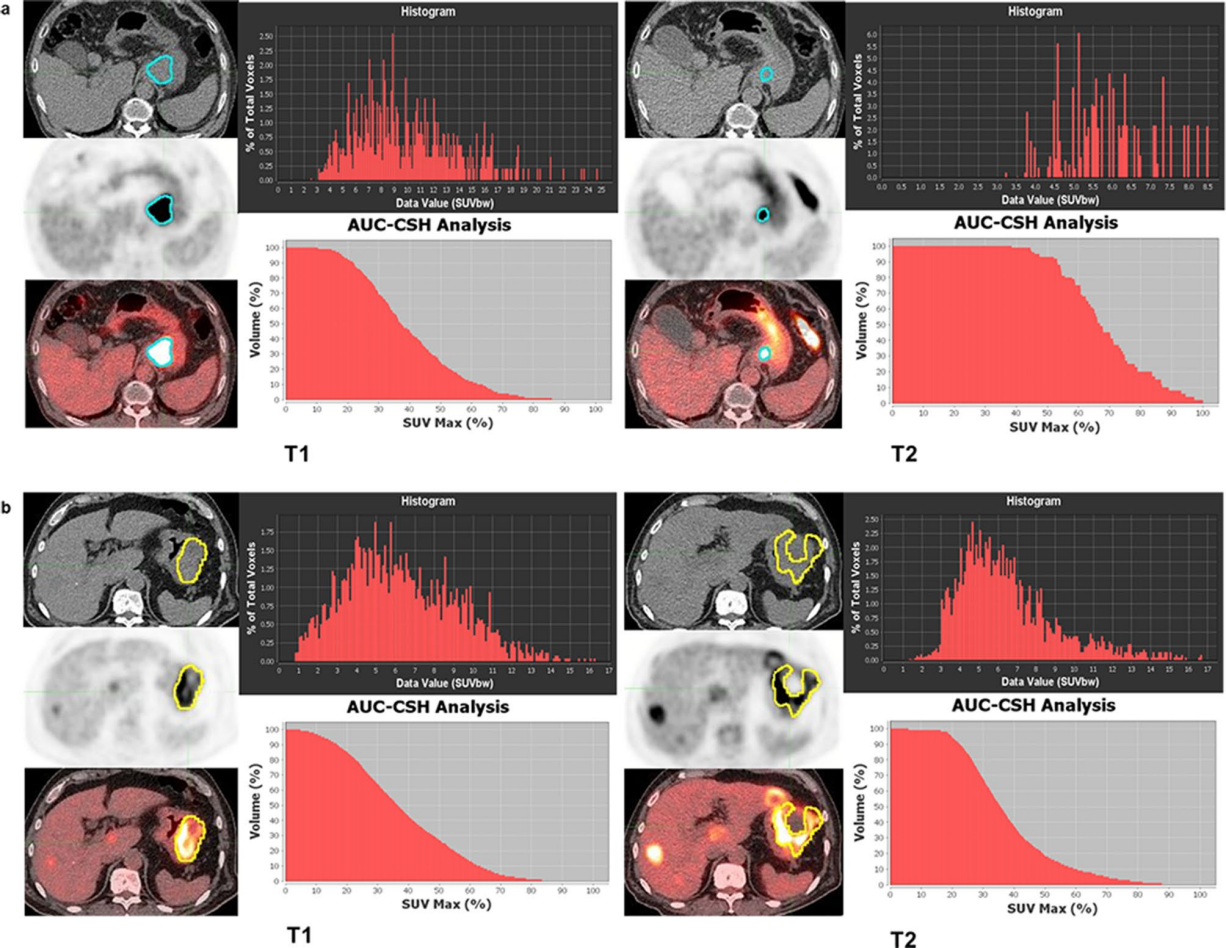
Representative cases showing clinical significance of metabolic ITH changes from [^18^F]FDG-PET-CT. Case (a) is a 74-year-old male patient with PFS of 19.5 months and OS of 19.8 months. The histogram and AUC-CSH showed decreased metabolic ITH after palliative chemotherapy. Case (b) is a 72-year-old male patient with PFS of 1.1 months and OS of 2.3 months, suggesting very poor prognosis. The histogram and AUC-CSH represent no significant change of metabolic ITH after palliative chemotherapy. *AUC-CSH *area under the curve of the cumulative standardized uptake value volume histogram, *[*^*18*^*F]FDG-PET-CT *[^18^F]fluoro-2-deoxy-d-glucose positron emission tomography-computed tomography, *ITH *intratumoral heterogeneity, *OS *overall survival, *PFS *progression free survival, *ROI *region of interest.
